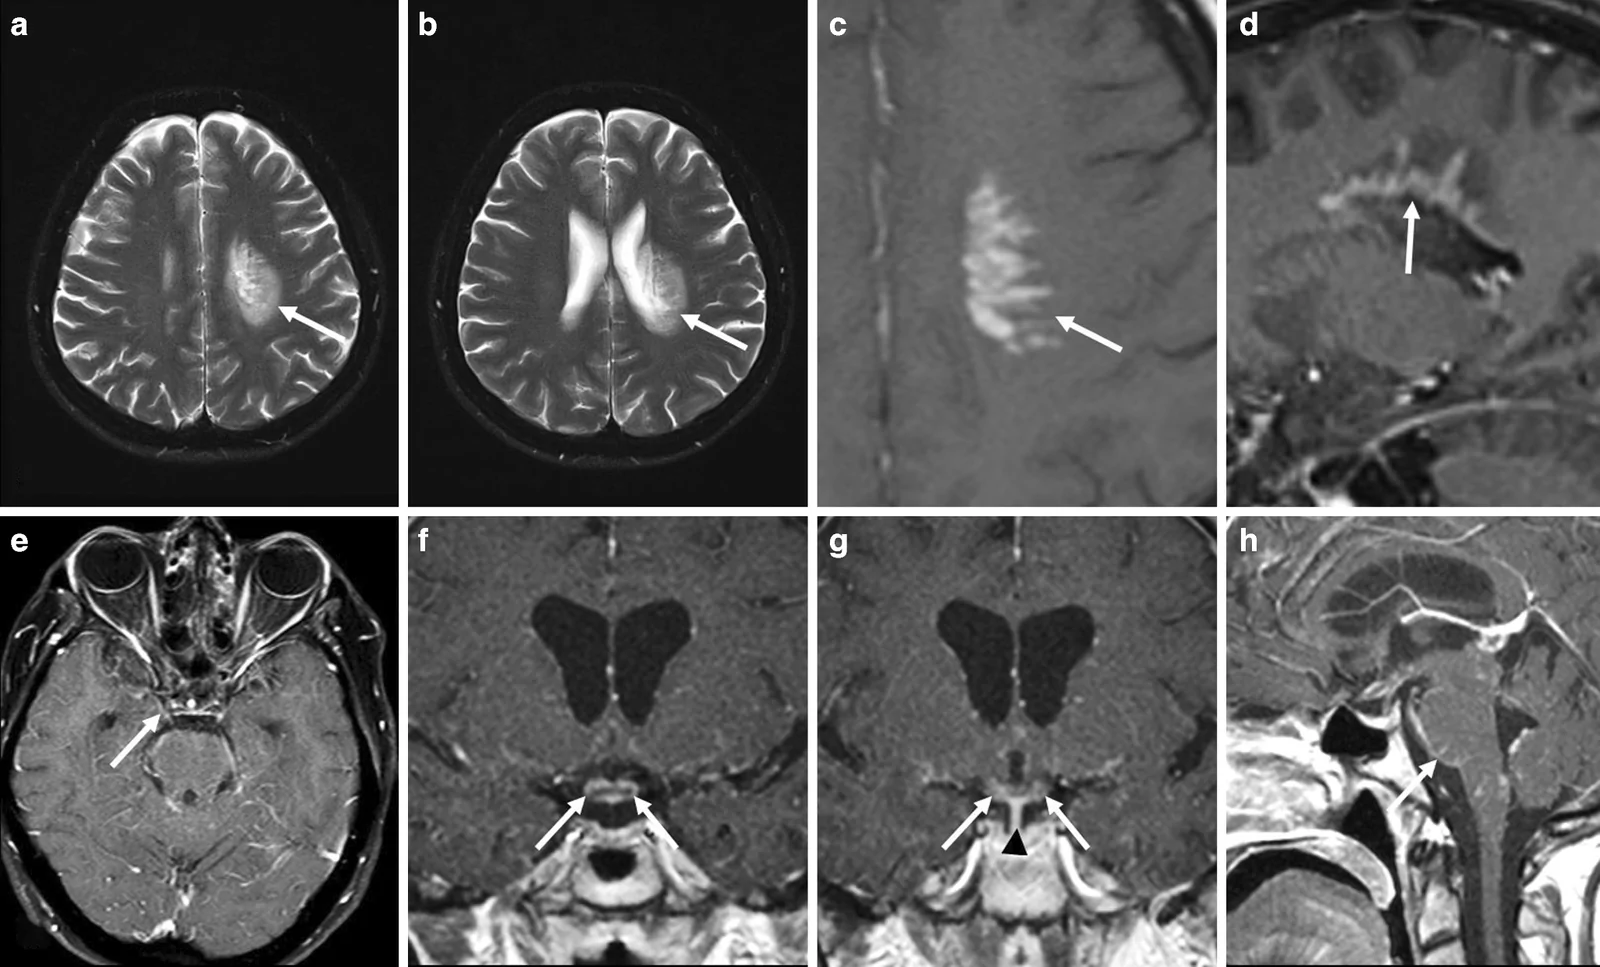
Neurosarcoidosis. a, b inhomogeneous hyperintense lesion on T2-weighted images (WI) in the left centrum semiovale (arrow) adjacent to the cella media in a 57-year old woman with progressive right sided hemiparesis; c, d distinct enhancement with angiocentric pattern (arrow) on T1 WI after contrast medium (CM) administration; e–h T1 WI after CM administration showing enhancement of the cranial nerves (e, arrow: oculomotor nerve; f, g optic nerve and chiasm, arrow), the pituitary stalk (g, black arrowhead) and the pontine perivascular spaces (h arrow)
